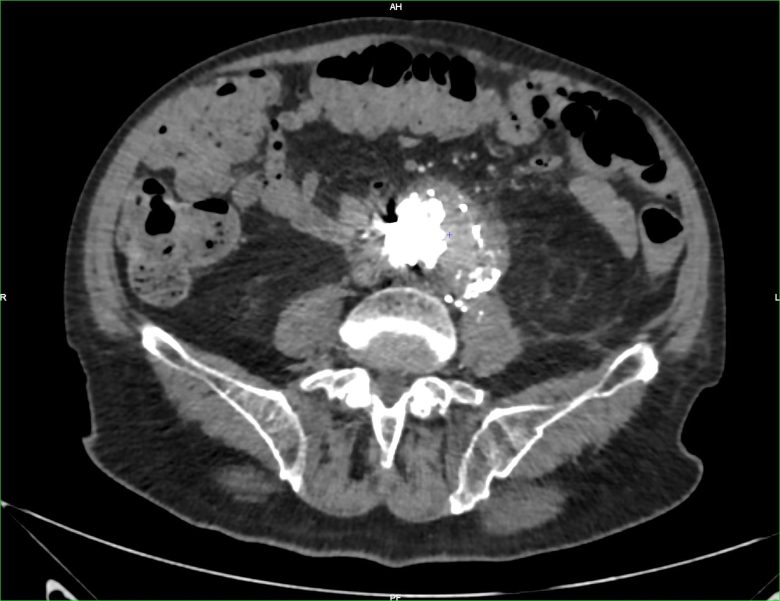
CT scan of the rupture with the modification of the aneurysmal sac on the left side.
